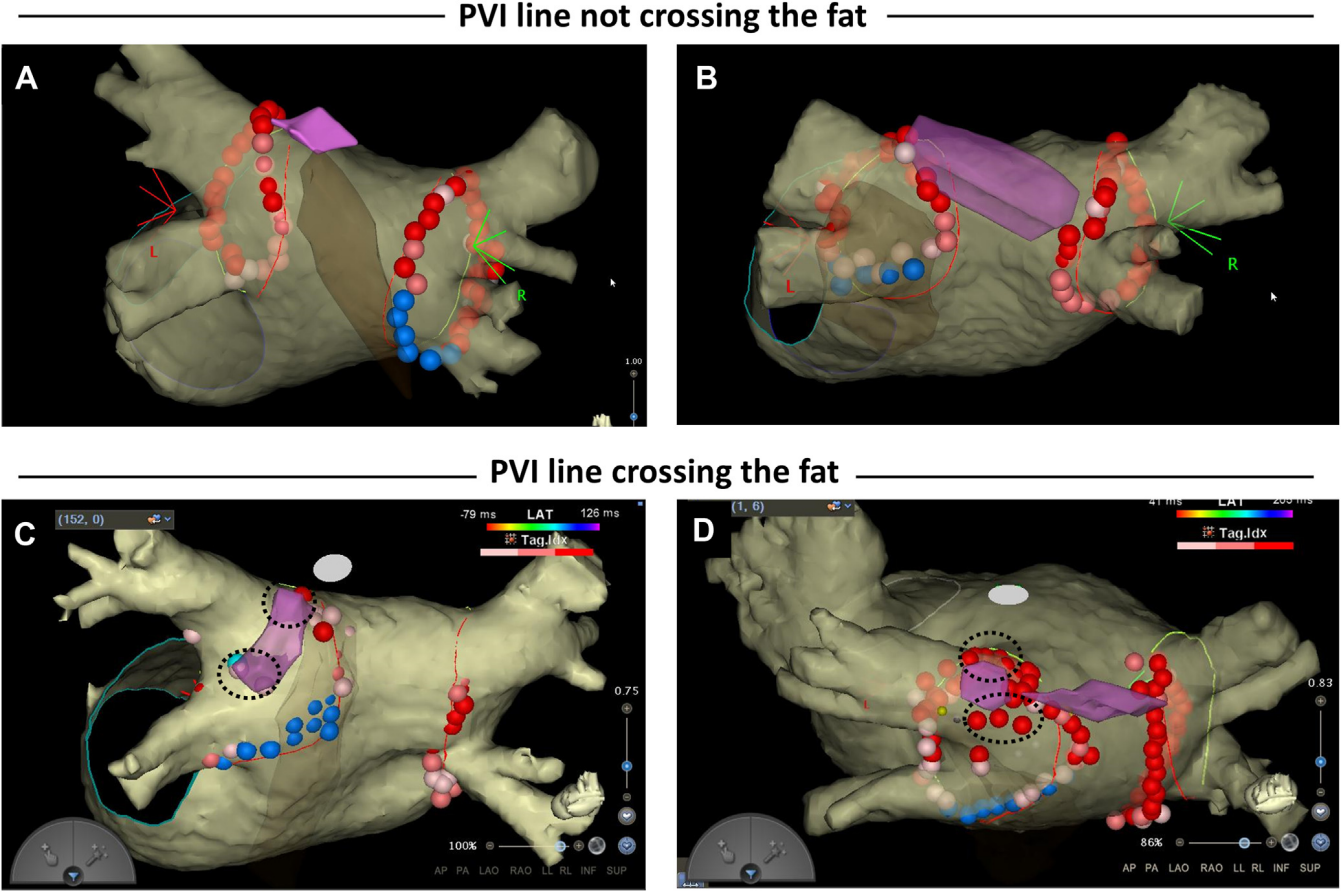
Representative PVI line in patients with fat on the LA roof. A: Patient with a focal fat region at the LSPV-LA junction. A modified PVI line that avoided fat achieved first-pass isolation without acute recurrence. B: Patient with a widely spread fat region between the LSPV and the RSPV. A modified PVI line avoiding fat was successfully created, resulting in first-pass isolation with no acute recurrence. C: Patient with a focal fat region extending from the LSPV-LA junction to the posterior carina. A spontaneous reconnection occurred after first-pass isolation. Reisolation was achieved by ablating the roof and posterior carina adjacent to the fat region (*dotted circle*). D: Patient with a widely spread fat region on the inner side of the LSPV. First-pass isolation was not achieved, requiring ablation of the inner side of the PV in front of and behind the fat region (*dotted circle*). The *pink area* represents the fat region identified using ICE. The *brown area* indicates the esophagus identified using ICE. ICE = intracardiac echocardiography; LA = left atrium/atrial; LSPV = left superior pulmonary vein; PV = pulmonary vein; PVI = pulmonary vein isolation; RSPV = right superior pulmonary vein.
